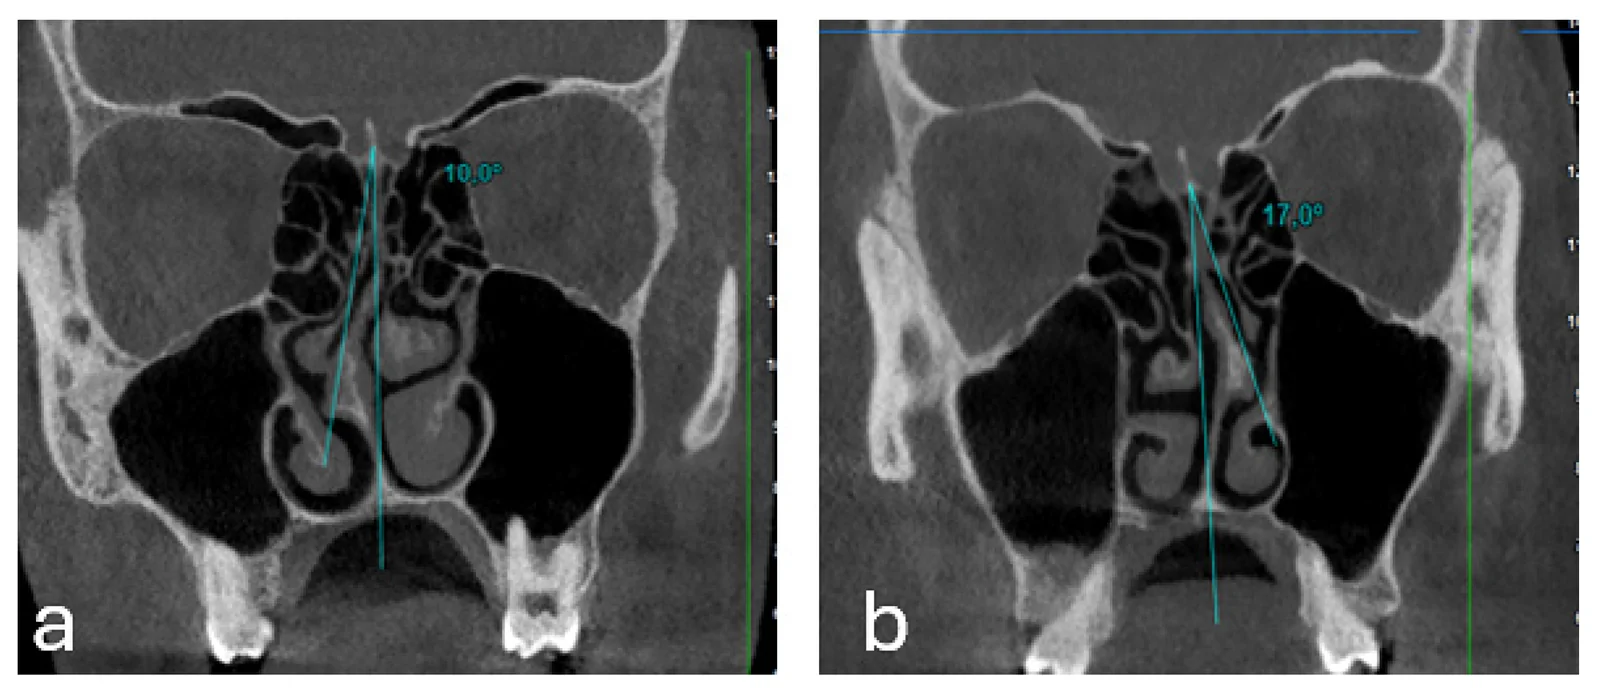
Representative coronal cone-beam computed tomography (CBCT) images showing nasal septal deviation (NSD) directions: (a) right-sided NSD and (b) left-sided NSD.
